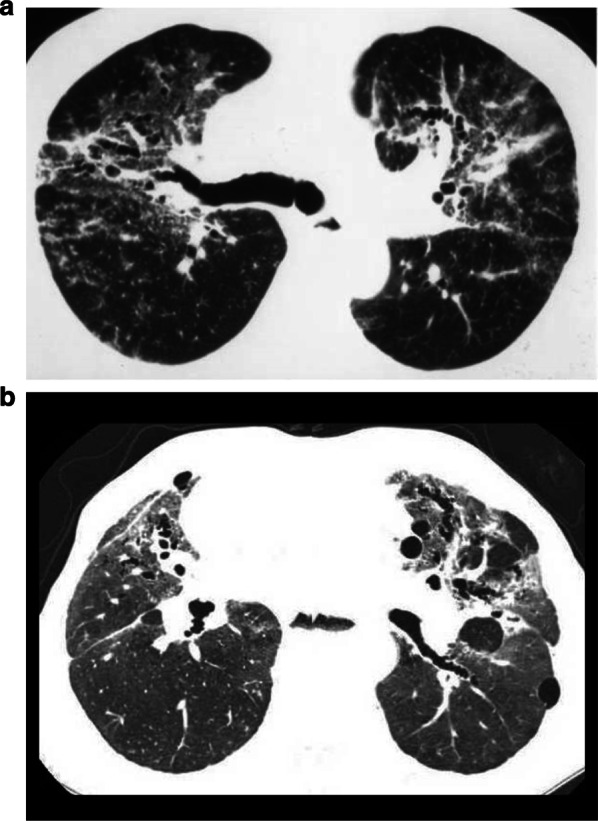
HRCT scans of pulmonary aluminosis. a 58-year-old man with pulmonary aluminosis. HRCT scan of pulmonary aluminosis mimicking sarcoidosis. Ground-glass opacities, small nodular opacities, and traction bronchiectasis are seen predominantly around the bronchovascular bundles. Nodules are located in both centrilobular and paralobular regions. b 52-year-old man with pulmonary aluminosis. HRCT shows traction bronchiectasis and ground-glass opacity predominantly in the upper lungs. Multiple bullae and centrilobular nodules are also seen
